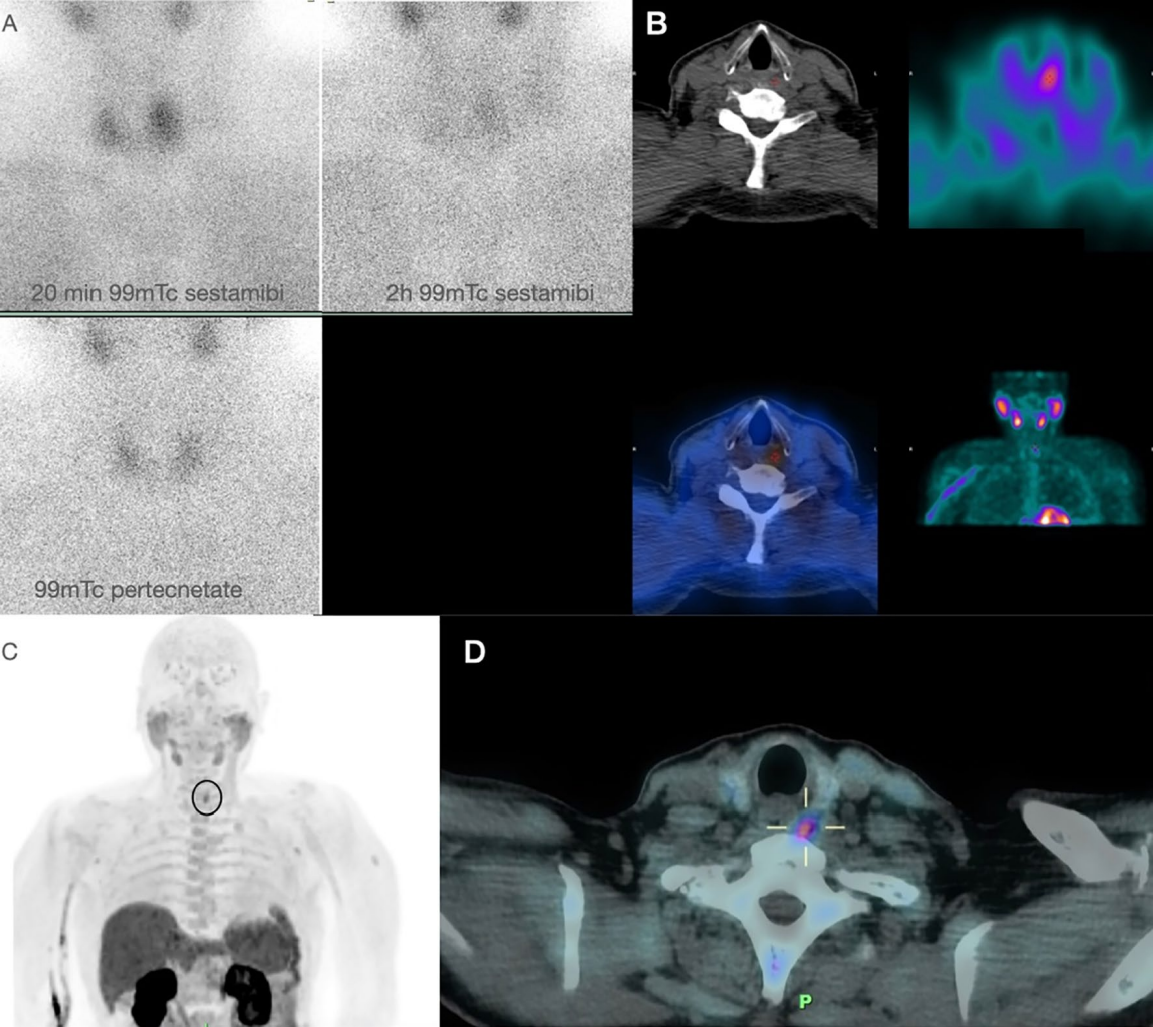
A Double tracer ^99m^Tc-sestamibi and ^99m^Tc pertechnetate in 71-year-old patient with primary HPT (PTH 226 pg/ml) and no evidence of enlarged parathyroid on ultrasonography and 4D CT; B SPECT/CT shows no findings likely to be an adenomatous parathyroid. C–D [^18^F]F-choline PET/CT MIP and axial images show the presence of small area of focal hyperfixation at the left upper parathyroid
